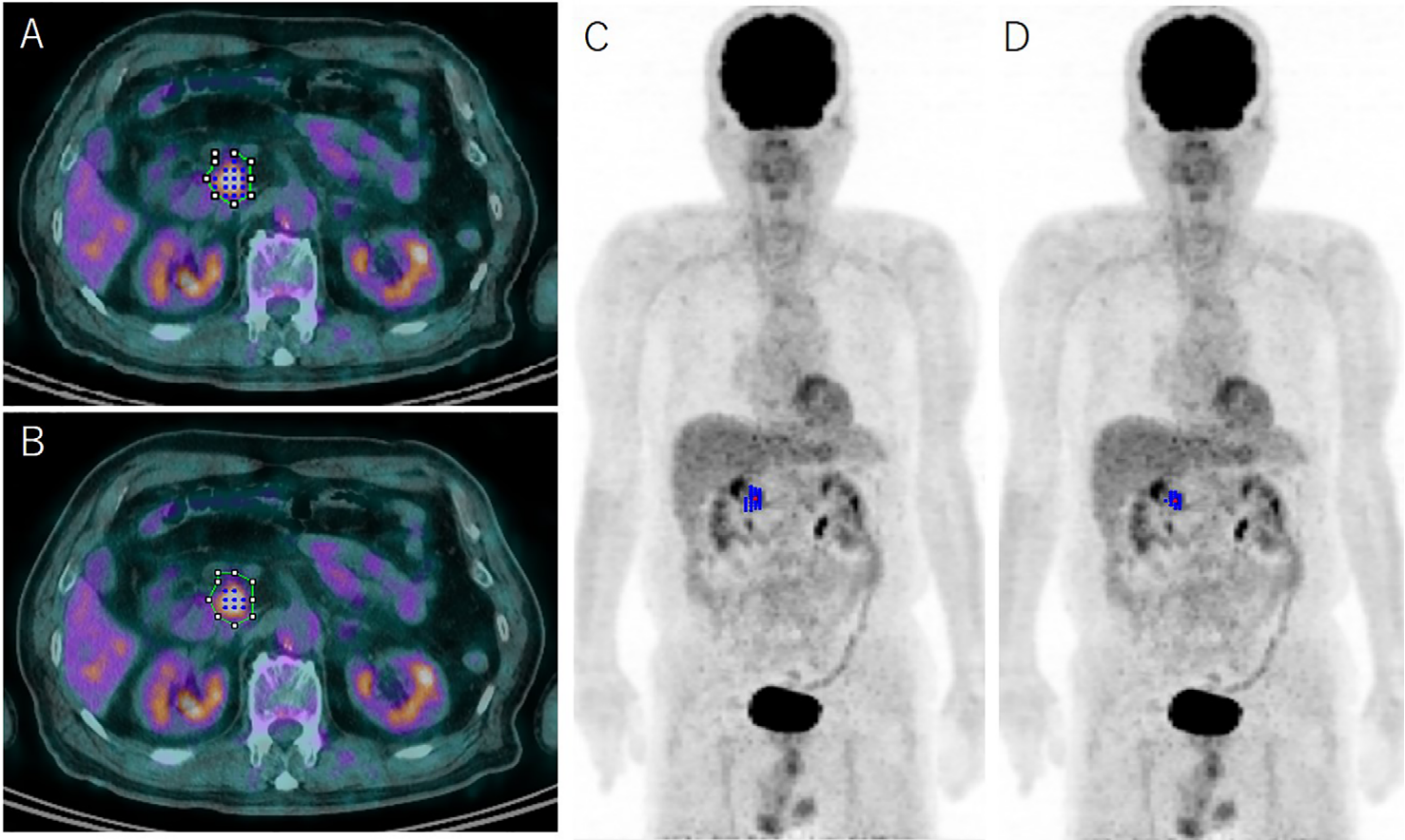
Representative case determined using the absolute 2.5 threshold method (A, C) and the relative 41% threshold method (B, D) with representative axial ^18^F-fluorodeoxyglucose-positron emission tomography (FDG-PET)/computed tomography fusion images and 3D reconstructed FDG-PET images. The green line on the axial images (A, B) indicates the initial volume of interest for determining metabolic parameters of pancreas ductal adenocarcinoma on FDG-PET; the blue dots and lines show the identical metabolic tumor volume (MTV) using the absolute 2.5 method (A, C) and the relative 41% method (B, D), respectively. The MTV is grossly underestimated using the relative 41% method compared with the 2.5 method.
